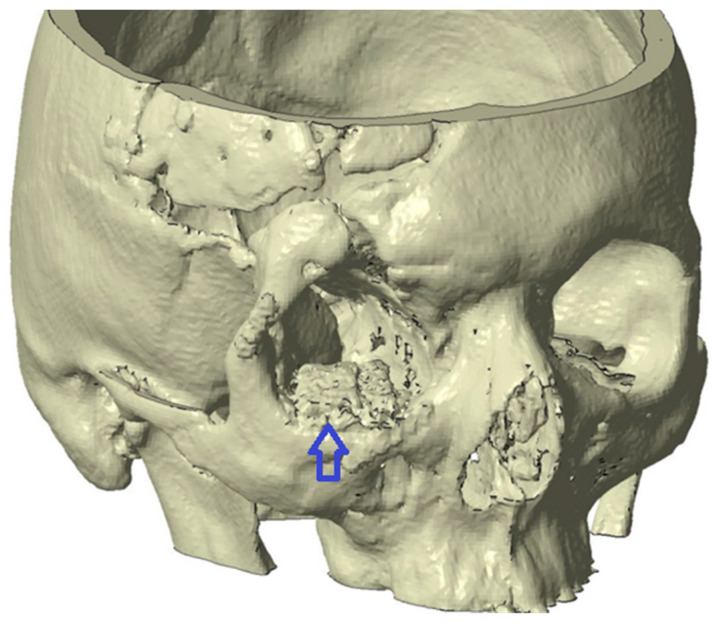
Three-dimensional reconstruction of the orbitomaxillary complex showing multiple overlapping titanium meshes (blue arrow) and secondary volume asymmetry compared with the contralateral side. Multiplanar CT analysis confirmed inferior displacement of the orbital contents and malar volume deficiency.
